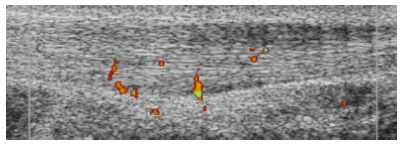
Ultrasound and Doppler examination showing a thickened Achilles tendon mid
